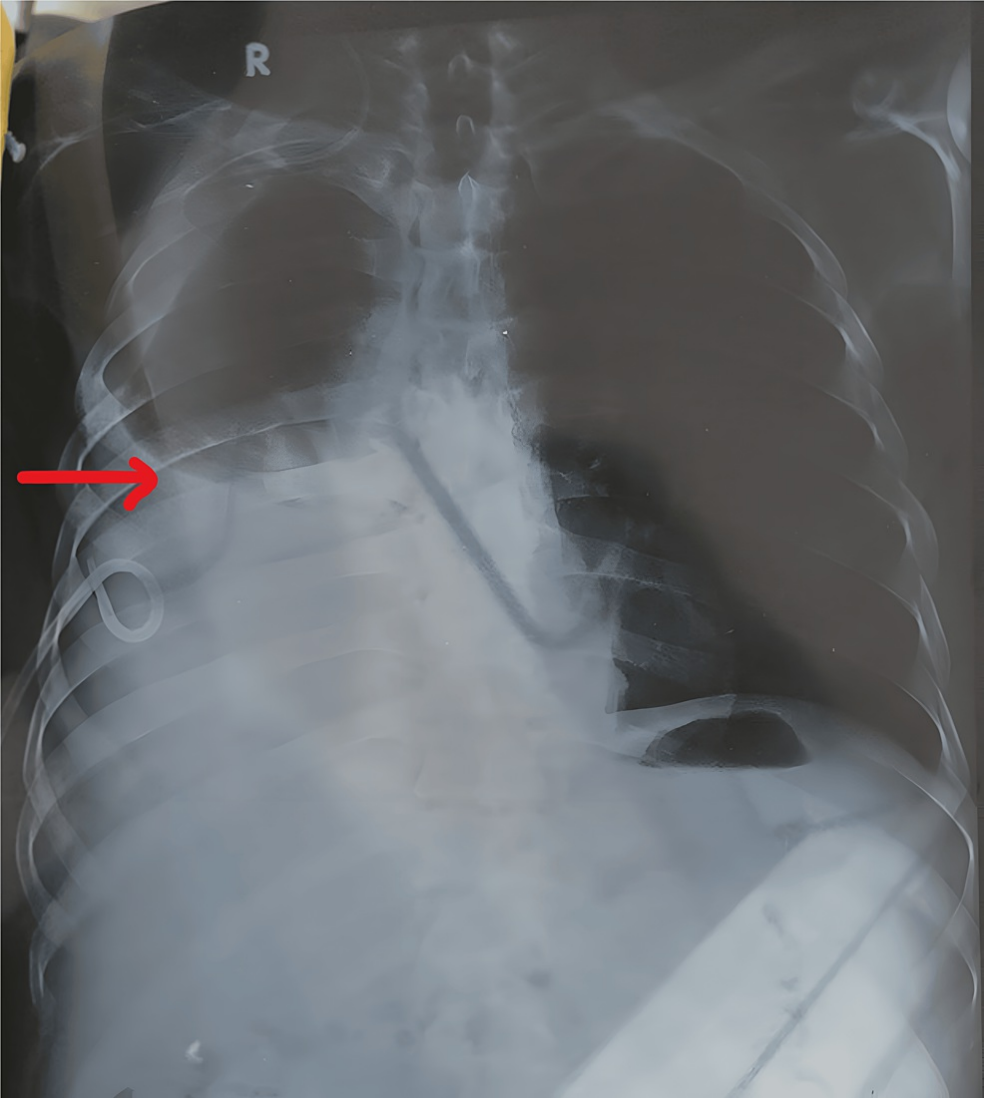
X-ray showing moderate pleural effusion on the right side. X-ray showing moderate right-side pleural effusion with pigtail catheter, and mild left-side pleural effusion showing blunting of costo-phrenic angle.
